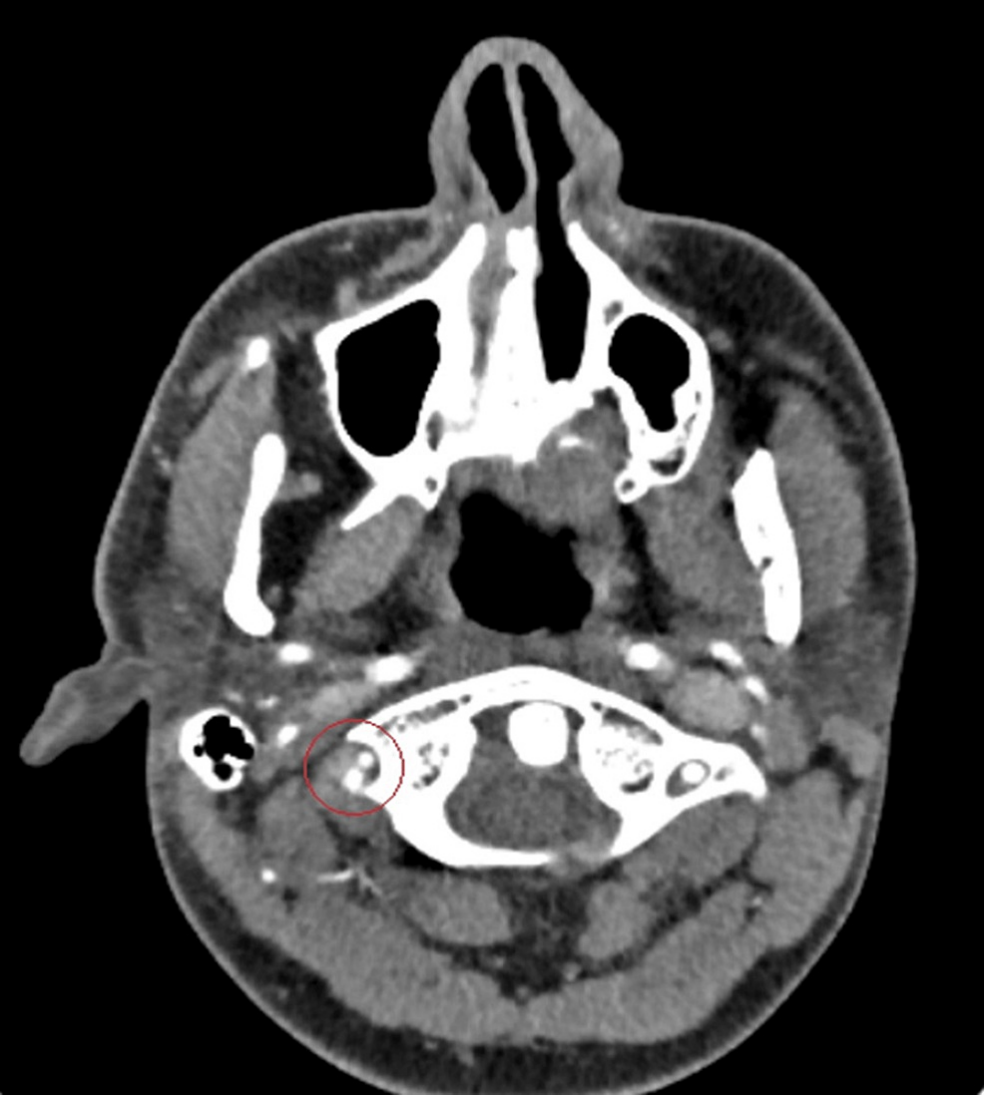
CTA head showing narrowing of the right vertebral artery (red circle) CTA: CT angiography
